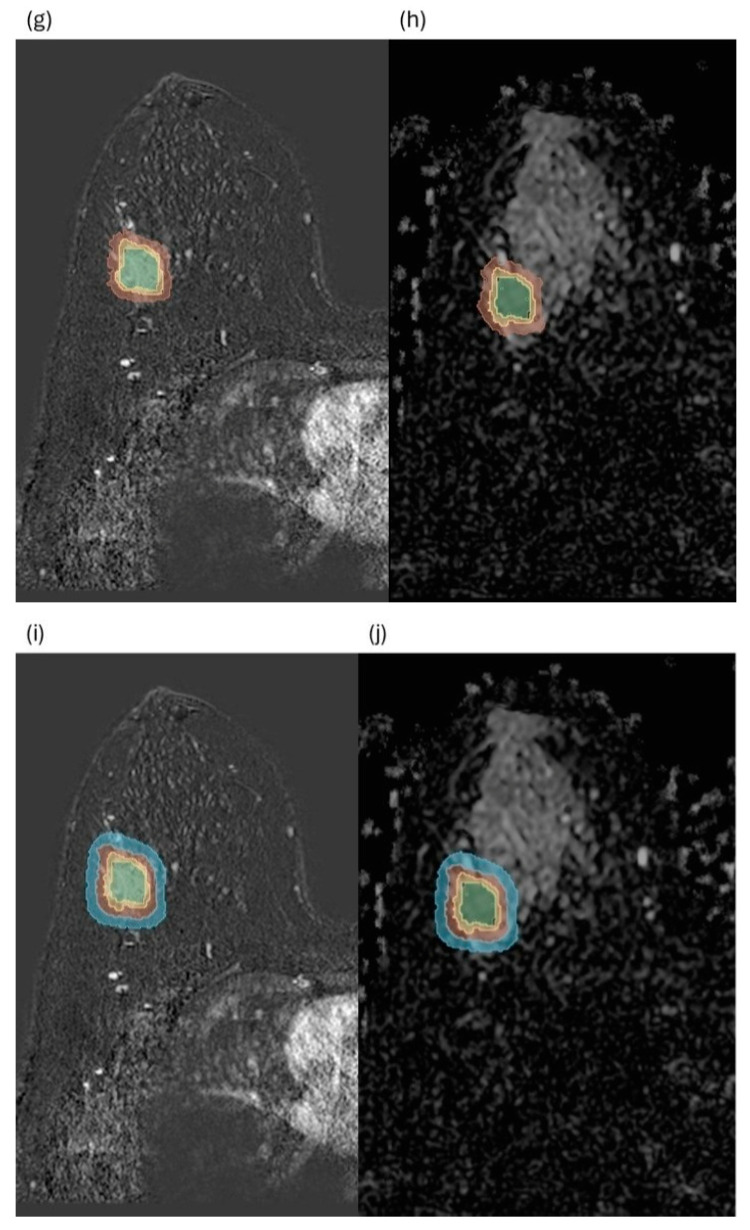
Representative example of intratumoral and peritumoral diffusion analysis using a segmentation-based multilayer concentric peritumoral shell model in invasive breast cancer. (a) Axial post-contrast T1-weighted image demonstrating the primary tumor. (b) Corresponding apparent diffusion coefficient (ADC) map. (c) Volumetric intratumoral segmentation overlaid on the post-contrast T1-weighted image (light green region). (d) Intratumoral region of interest overlaid on the ADC map (light green contour). (e) First peritumoral shell (0–2 mm) segmentation overlaid on the post-contrast T1-weighted image (yellow rim). (f) Corresponding ADC map showing the first peritumoral shell (0–2 mm). (g) Second peritumoral shell (2–5 mm) segmentation overlaid on the post-contrast T1-weighted image (orange rim). (h) Corresponding ADC map showing the second peritumoral shell (2–5 mm). (i) Third peritumoral shell (5–10 mm) segmentation overlaid on the post-contrast T1-weighted image (blue rim). (j) Corresponding ADC map showing the third peritumoral shell (5–10 mm).
